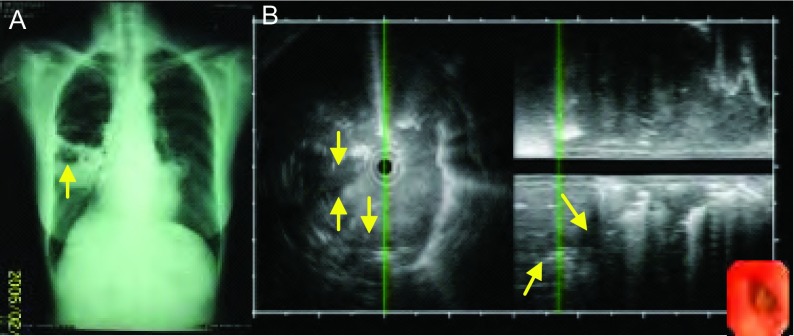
女，74岁，咳嗽、咳痰、发热10天，肺脓肿。A：胸片见右肺中叶和左肺舌叶片状阴影，内见空洞（箭头）；B：支气管镜下见右中叶外侧段粘膜充血，支气管内超声探查其远端肺实质见低回声病灶，边界欠清晰，内部回声不均匀，局部可见不规则更低回声区（箭头），考虑为坏死空腔。 Female, 74 years old, cough, and expectoration of sputum for 10 days, diagnosed pulmonary abscess. A: Chest film shows large shadow at the right middle lobe and left lingular lobe, cavity can be seen inside (at the arrow tip); B: Bronchoscope shows mucous hyperemia at the segment of right-middle lobe, endobronchial ultrasound at the distal lung parenchyma shows a low echo lesion with fuzzy border, heterogeneous echo, more lower echo area can be found in area (at the arrow tip), regarded as necrotic cavity.
